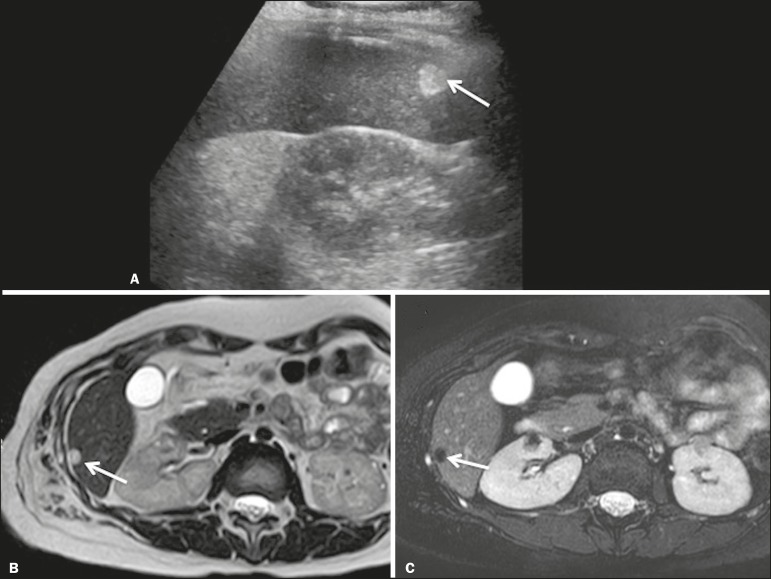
Hepatic lipoma in a 15-year-old female with tuberous sclerosis. A: Ultrasound of the liver, showing a hyperechoic circumscribed nodular lesion (arrow). B: T2-weighted magnetic resonance imaging sequence showing a small, round lesion with a clearly hyperintense signal (arrow) and evident signal loss following fat suppression (arrow in C), behavior similar to that of subcutaneous tissue, proving that the lesion had a fat component and confirming the diagnosis of lipoma.
